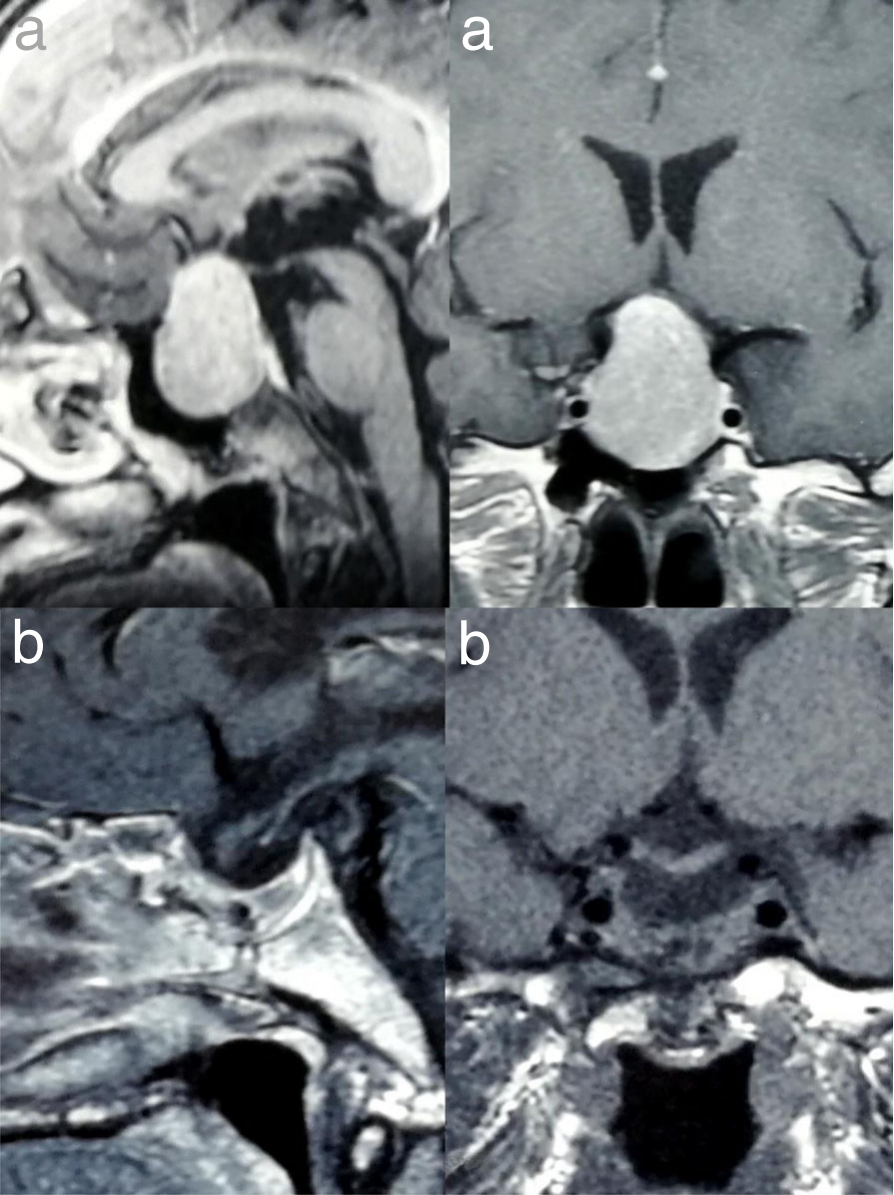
Coronal and sagittal MRI images of a patient who underwent a STT approach a. MRI image before surgery, showing a large pituitary adenoma extending suprasellar area and compressing the optic chiasm upward, b. MRI image 6 month later STT surgery showing fat grafts in the sella turcica and sphenoid sinus. The diaphragm sellae has descended into the sellar cavity and revealed complete disappearance of the tumor in the sellar and suprasellar region
